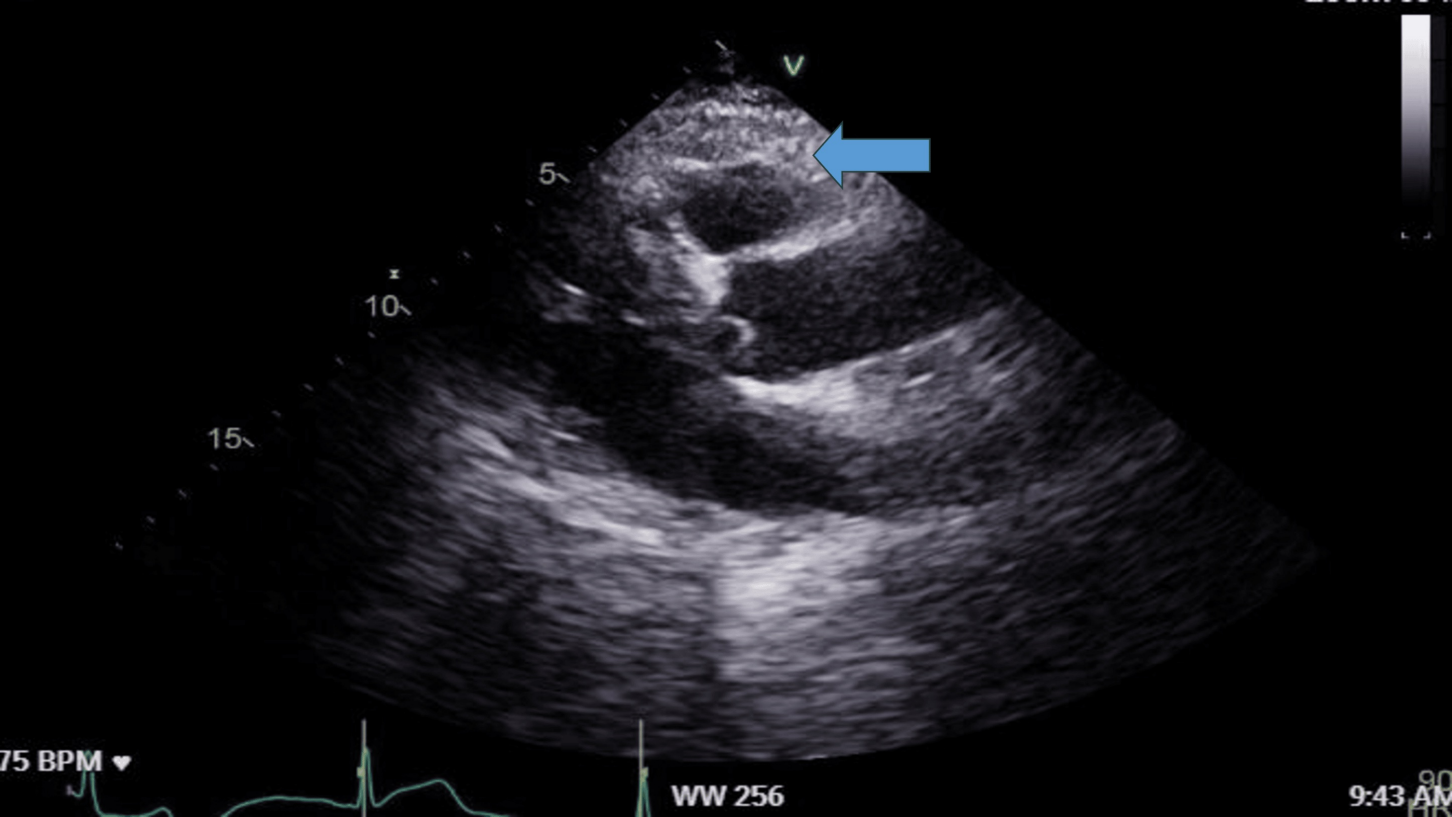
Normal Echocardiogram Arrow indicates normal RV RV, Right ventricle
